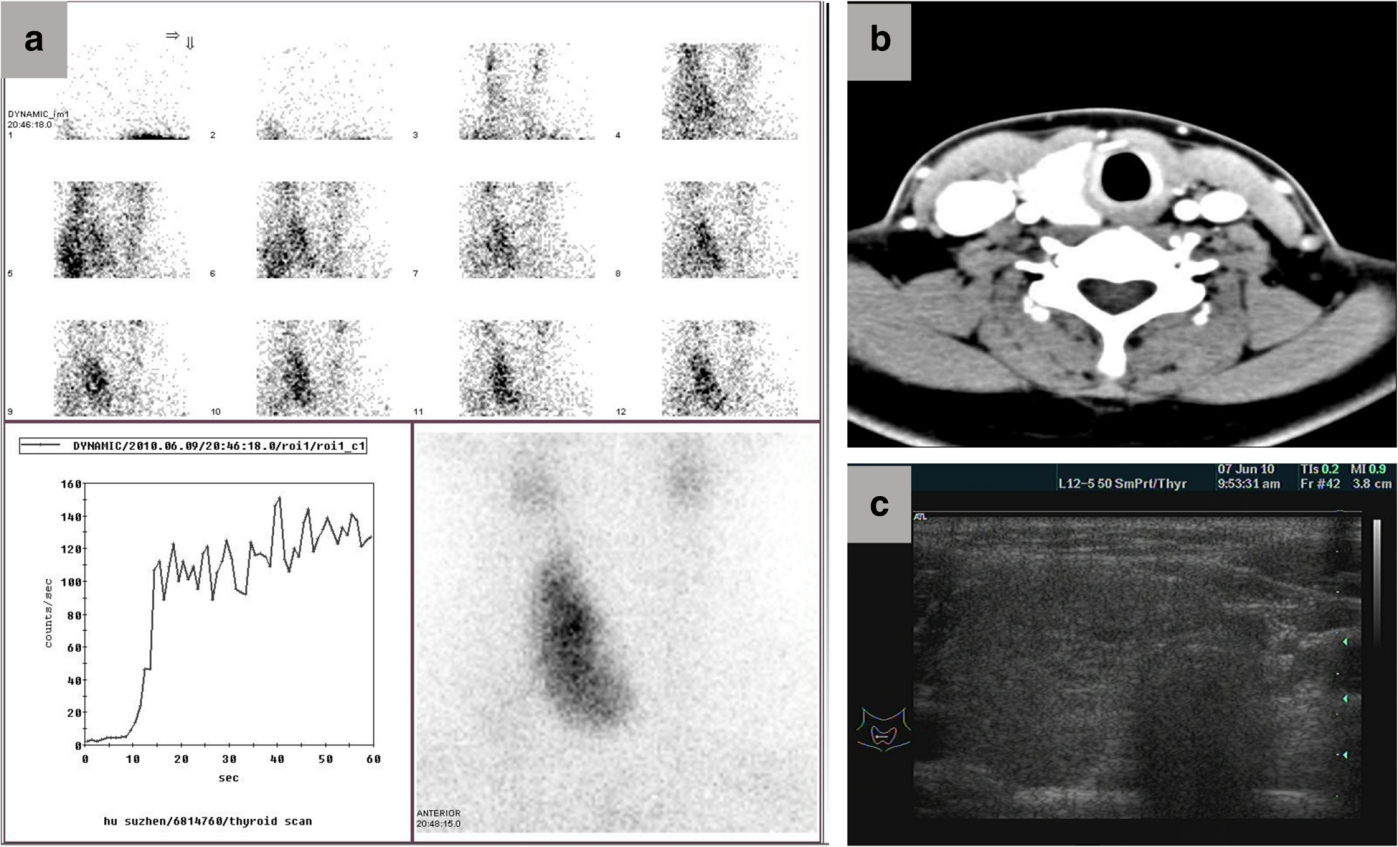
Augmentation of right lobe and absence of left lobe of thyroid a. Thyroid radionuclide imaging, b. CT scanning, c. Ultrasonic examination
